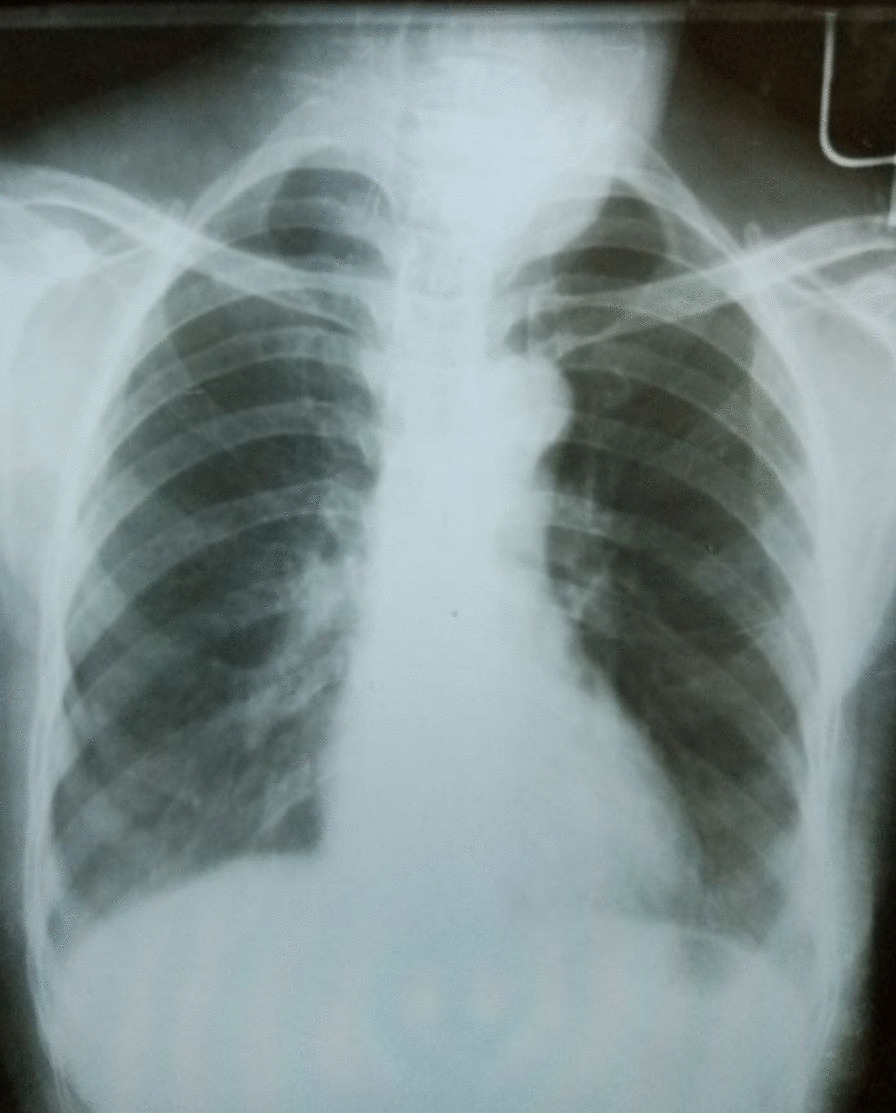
Chest X-ray revealing normal-looking lung parenchyma and thyroid mass
